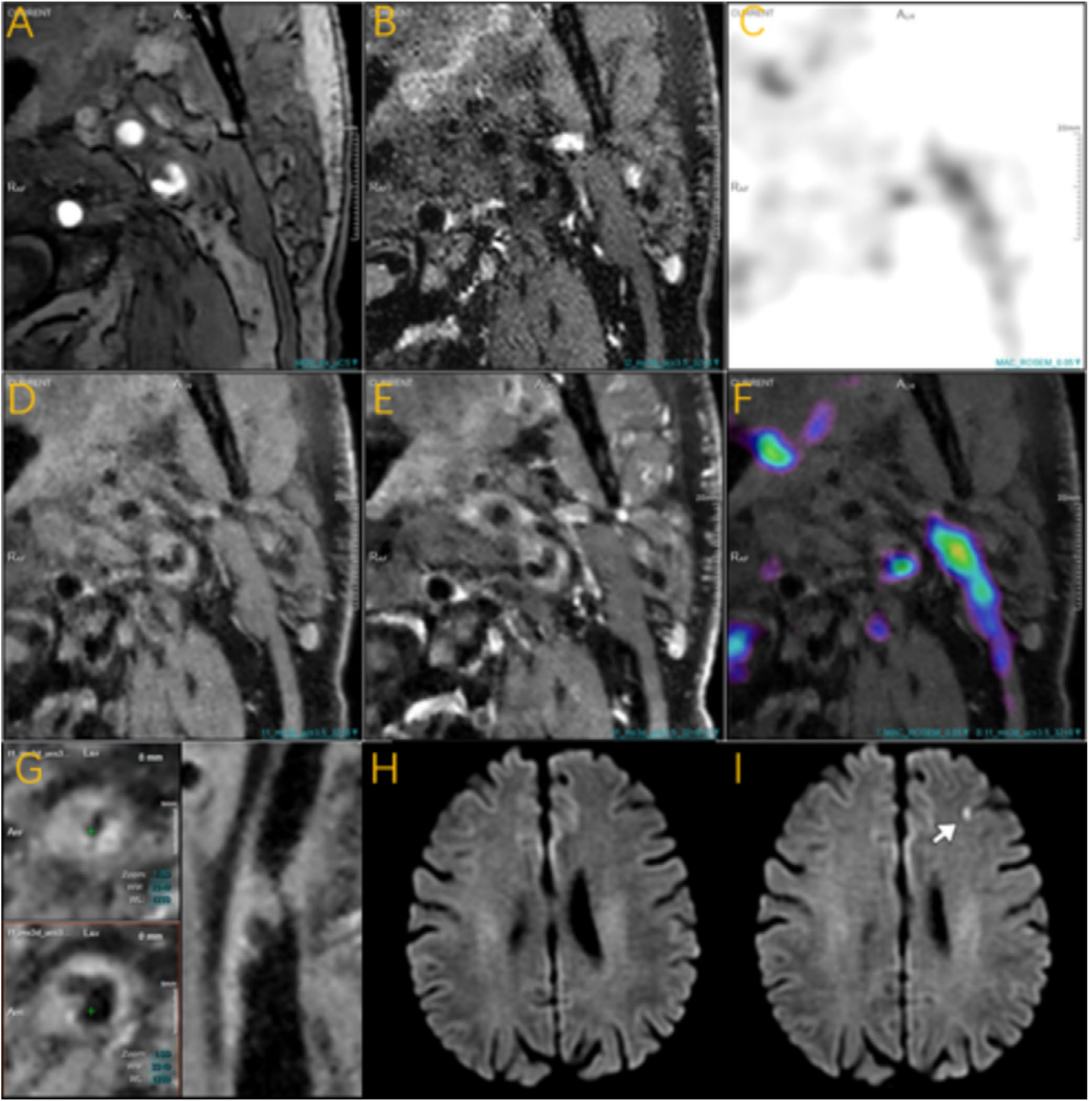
A male ages 60–70 years old with severe carotid stenosis treated by CAS. Images from A to sequentially demonstrate the carotid atherosclerotic plaque in this patient using different PET/MR sequences ((A) Time‐of‐flight MR angiography (TOF‐MRA); (B) T2‐weighted imaging (T2WI); (C) PET metabolic map; (D) T1‐weighted imaging (T1WI); (E) T1‐weighted contrast‐enhanced imaging (T1+C); (F) Fusion of T1WI and PET metabolic map; (G) Curved planar reformation (CPR) reconstruction; (H) Pre‐treatment brain diffusion‐weighted imaging (DWI); (I) Post‐treatment brain diffusion‐weighted imaging (DWI)), The surface of the plaque is irregular, showing a lipid‐rich necrotic core and ulceration, consistent with a Type VI—complex plaque in the AHA‐LT classification. The SUVmax is 3.16 and the TBR is 3.67, indicative of severe uptake. DWI of the brain before CAS (H) shows normal findings, DWI of the brain after CAS (I) at the same level shows new ipsilateral ischemic lesion in the left frontal lobe (white arrow).
